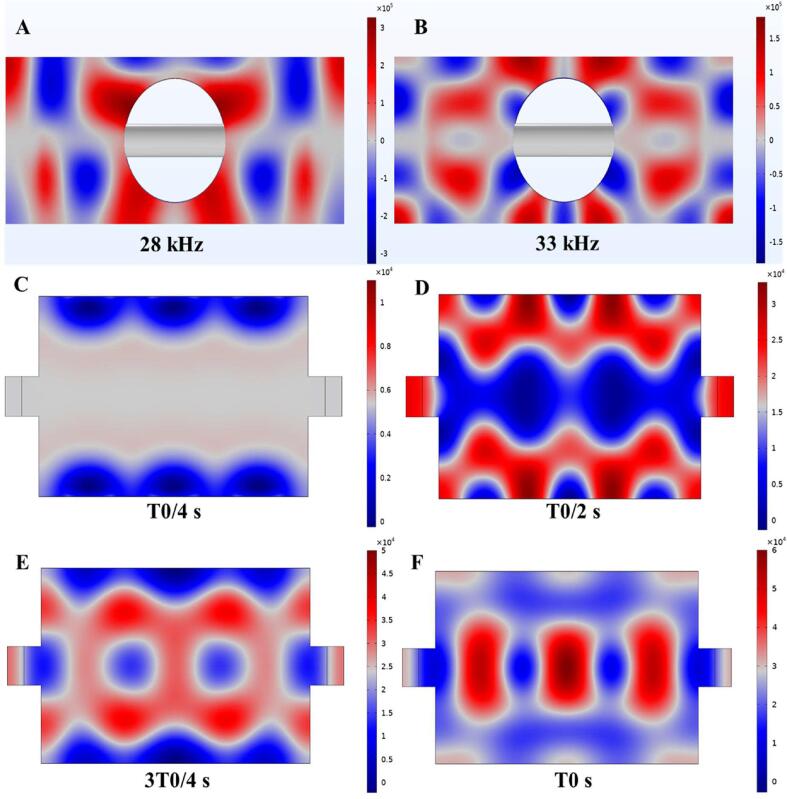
Absolute sound pressure (Pa) distribution under different ultrasound frequency modes. Single frequency ultrasound mode (A, B). Dual frequency ultrasound mode (C - F).
